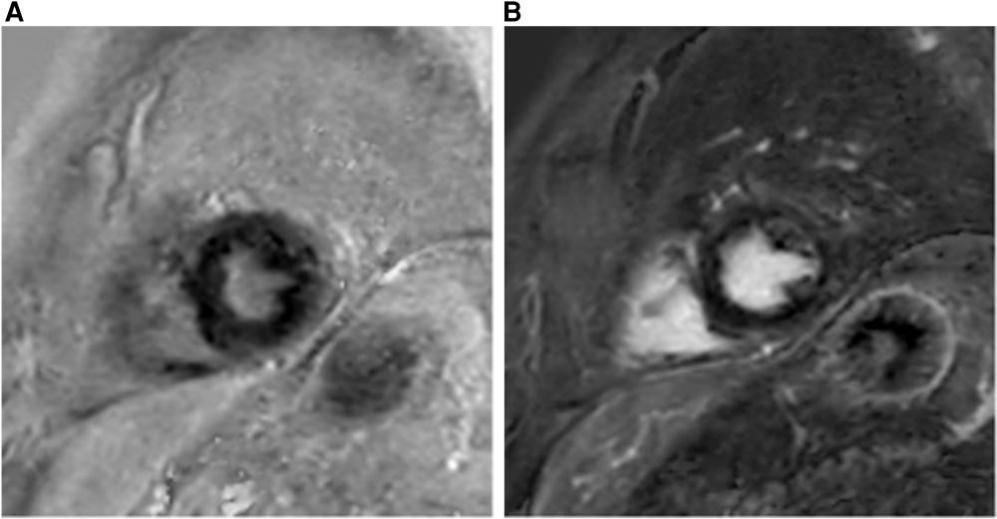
Baseline cardiac MRI in midventricular short axis view during initial hospitalization and at follow-up without evidence of LGE (MRI, magnetic resonance imaging; LGE, late gadolinium enhancement).
